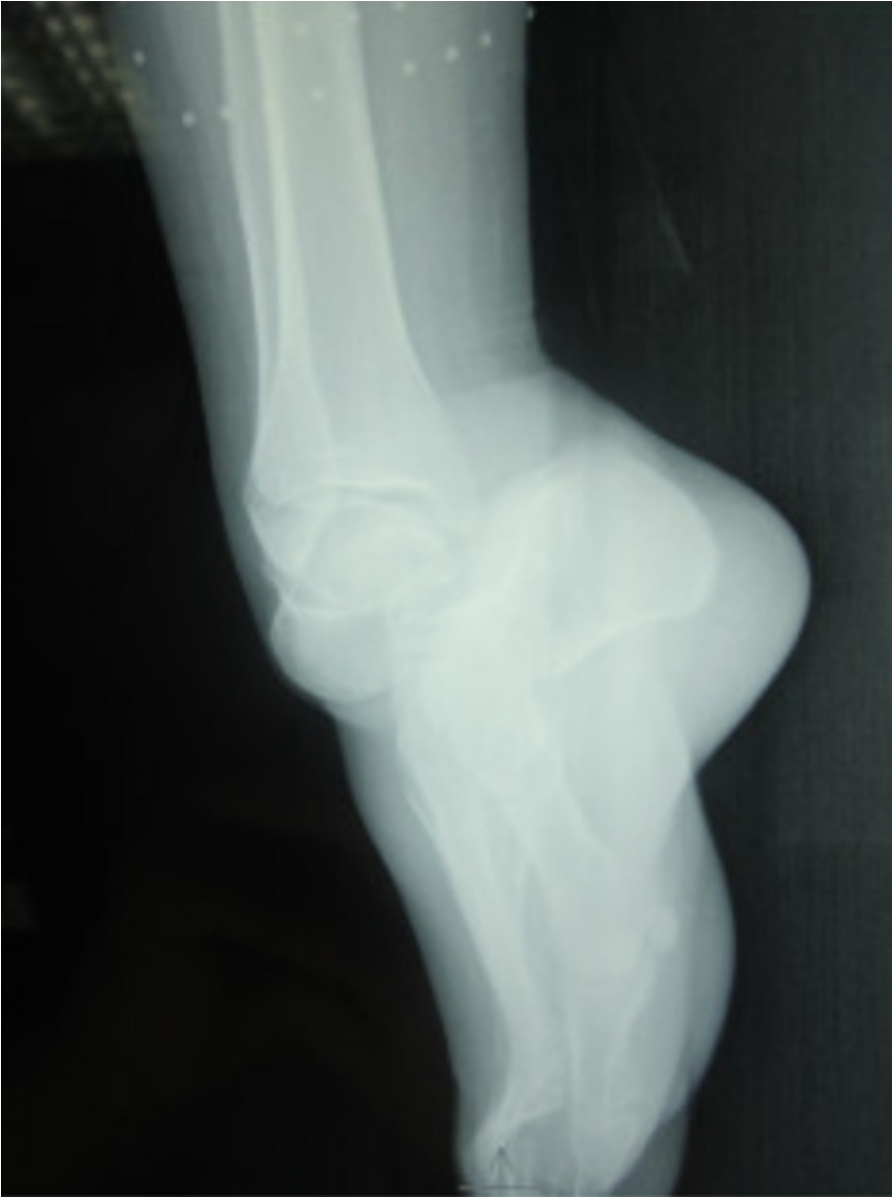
Lateral radiograph of the ankle showing medial subtalar dislocation without fractures.
